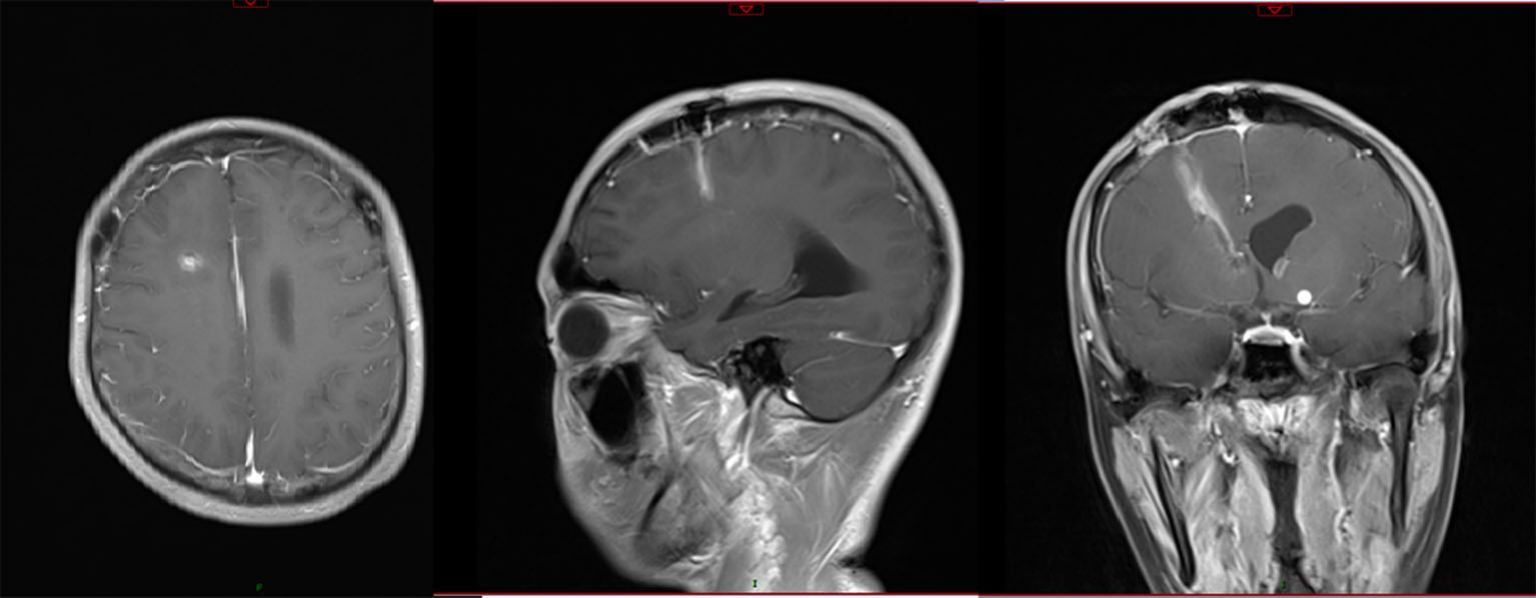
Enhanced MRI scanning after treatment with the new regimen for 14 days showed that the abscess had disappeared, with little enhancement along the surgical path.
